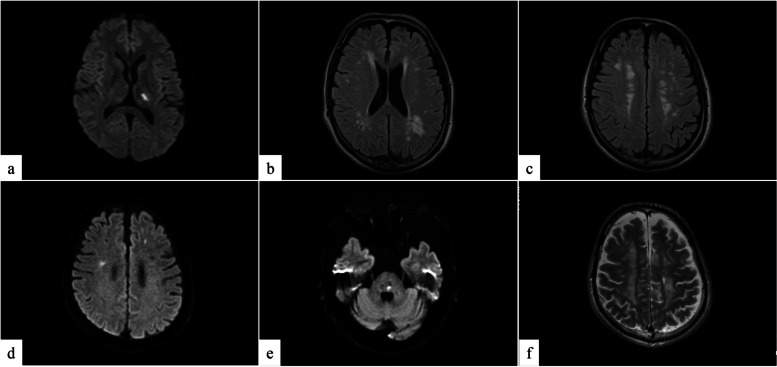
Magnetic resonance imaging. a Diffusion-weighted scan and (b, c) fluid-attenuated inversion recovery (FLAIR): Images revealed a new left internal capsule infarction and multiple asymptomatic brain infarctions at the age of 42 years. d, e Diffusion-weighted scan and (f) T2-weighted scan: the last images of consecutive episodes at the age of 48 years; new infarctions in the bilateral cerebral hemispheres and left pons were detected. There were wide-spread periventricular high-intensity lesions in the parietal cerebral area with different time phases
